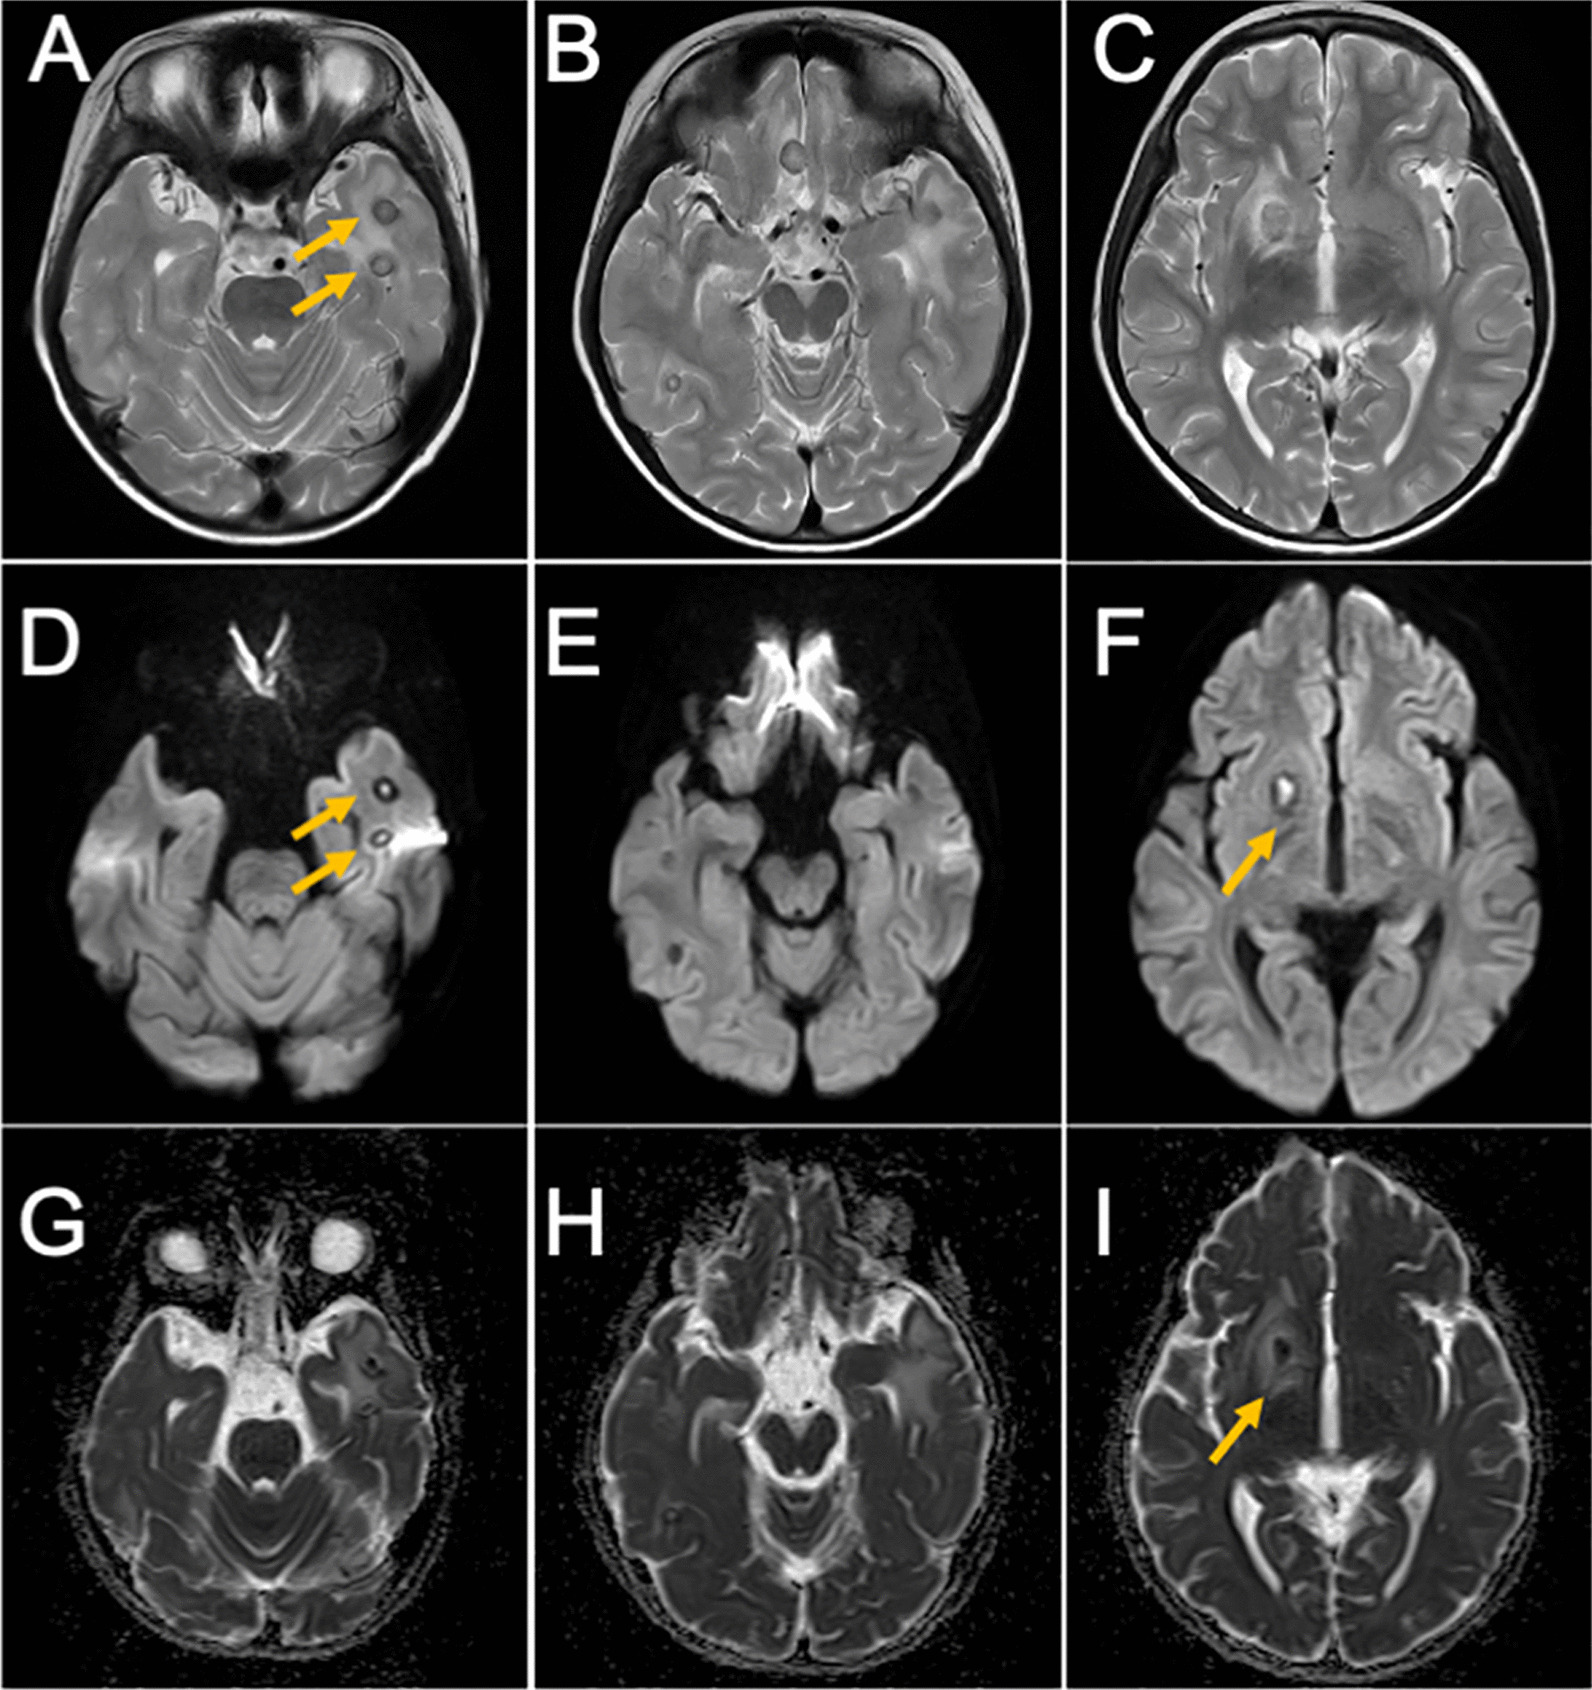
MRI obtained on November 11, 2020. T2-weighted image (A, B, and C), diffusion-weighted image (D, E, and F), ADC map (G, H, and I). Yellow arrows indicate brain abscesses
